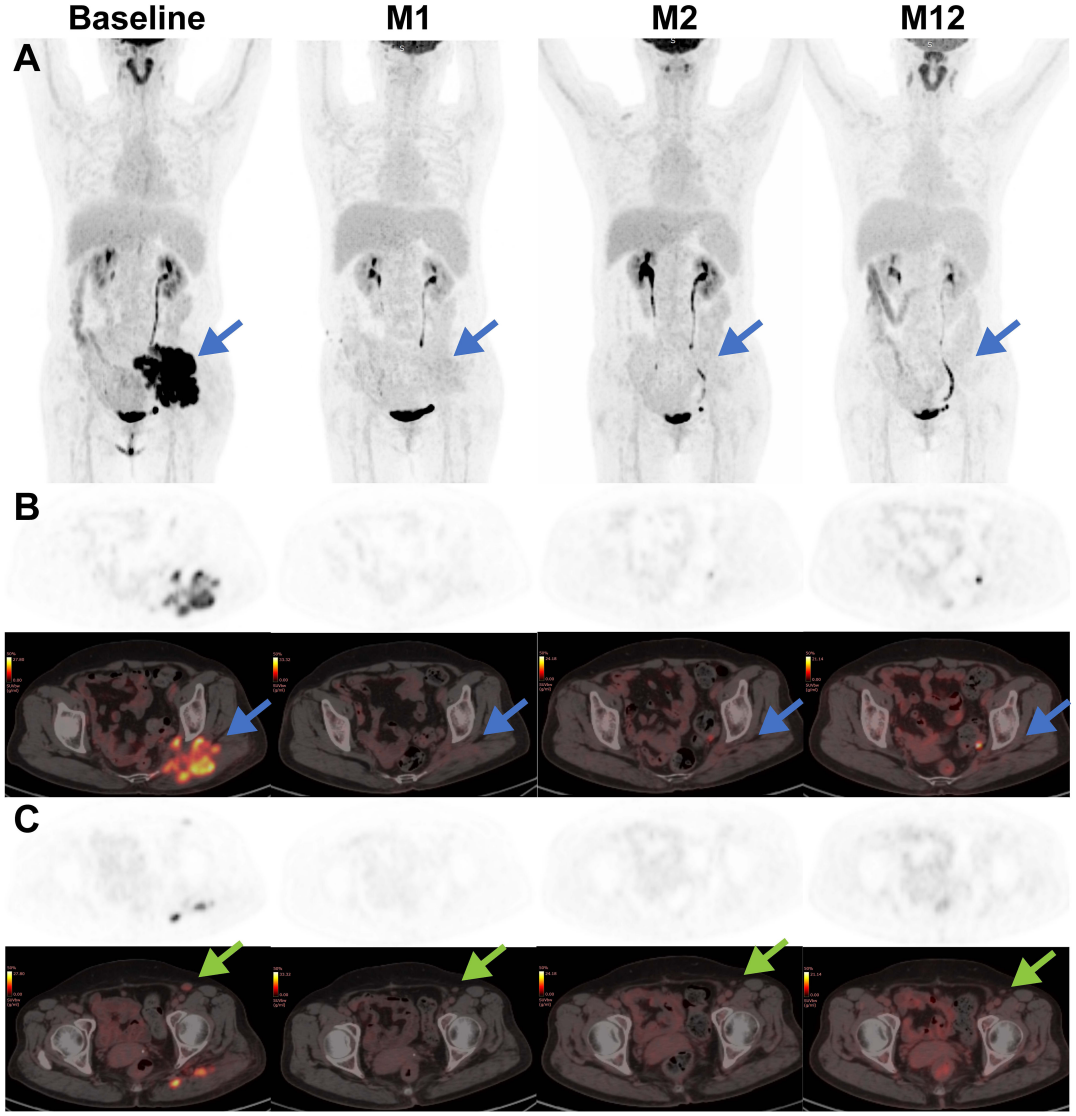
Representative coronal-view and axial-view images of serial 18F-FDG PET/CT scans before and after CAR T treatment. The baseline PET-CT shows a bulky mass (blue arrow) in the left buttocks with SUVmax of 30 and an enlarged left inguinal lymph node (green arrow) with SUVmax of 5.6. After CAR T infusion, the lymphoma lesions showed a rapid decrease in metabolic activity and a slow reduction in size, supporting the conclusion of complete remission. (A) Maximum intensity projection PET images; (B) axial images of lymphoma lesion in the left buttocks; (C) axial images of enlarged left inguinal lymph node.
